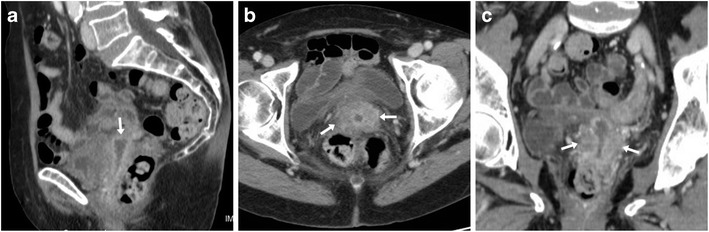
Expected CT appearance 4 days after uncomplicated vaginal hysterectomy (VH) performed to treat genital prolapse (GP), in an 80-year-old female suffering from abdominal distension, vague pelvic pain and low-grade fever. Multiplanar contrast-enhanced images (A–C) show a moderately distended vagina (arrows) with loss of the usual transverse configuration, circumferential mural thickening and marked, uniform mucosal enhancement reflecting diffuse vaginal oedema. The patient was discharged without any additional treatment (Partially reproduced from open access ref. no [58])
